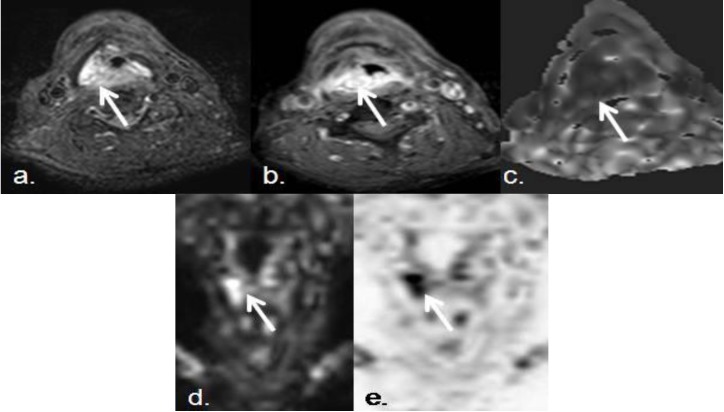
Seventy two year old male, with squamous cell carcinoma of the right piriform sinus.Despite his small size, this malignant lesion associated marked diffusion restriction. (a,b) Pre- and postcontrast axial SPIR TSE T1-weighted images show a small enhancing and infiltrating lesion in right piriform sinus (white arrows); (c) Axial ADC map, (d) DWIBS coronal MIP, and (e) DWIBS inverted MIP (pseudo-PET) show high increased signal in DIBWS sequence and decreased signal in ADC map, that confirms diffusion restriction of the lesion (ADC: 0.8 × 10^−3^ mm^2^/s). DWI and ADC can be considered in routine clinical practice as complementary sequences to anatomical ones, in order to increase diagnostic accuracy.
